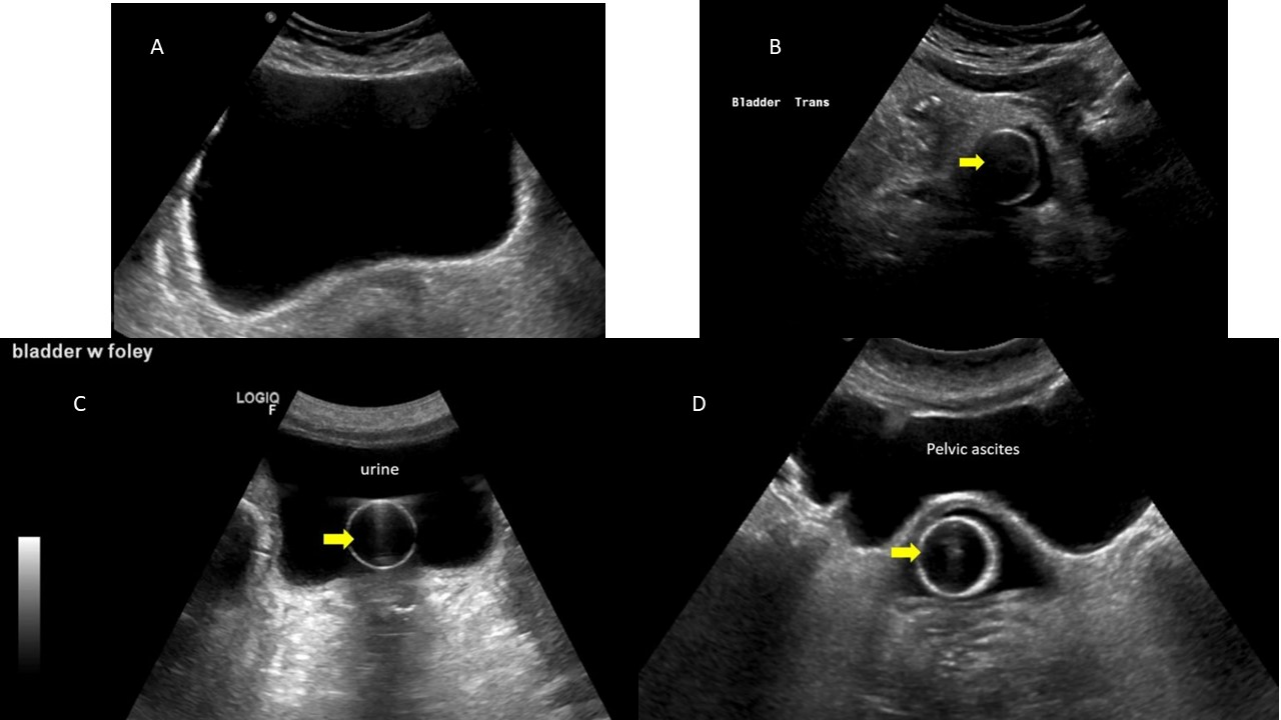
Bladder ultrasound. A) Normal transverse ultrasound of a full bladder. B) Decompressed bladder around an indwelling urinary catheter. Notice a small amount of hypoechoic urine around the catheter which is normal. C) Indwelling urinary catheter within a non-empty bladder. Note there is still a lot of anechoic urine within the bladder. This measured as >200 mL indicating improper drainage of urine. The indwelling urinary catheter was replaced and urinary flow resumed. D) Pelvic ascites mimicking bladder. Ascites is a common false positive for bladder scanners which mistake it for urine. On ultrasound we see the indwelling urinary catheter surrounded by a decompressed bladder (arrow). Superficial to this, one can see pelvic ascites interdigitating among abdominal viscera and tracking into the paracolic gutters. Images courtesy of Abhilash Koratala. Renalfellow.org/pocus-gallery. Used with permission.
